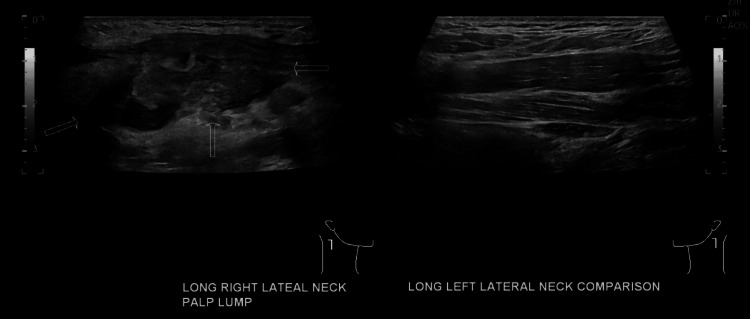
Ultrasound comparing both sides of the neck. Arrows indicate lesion on the right neck.
